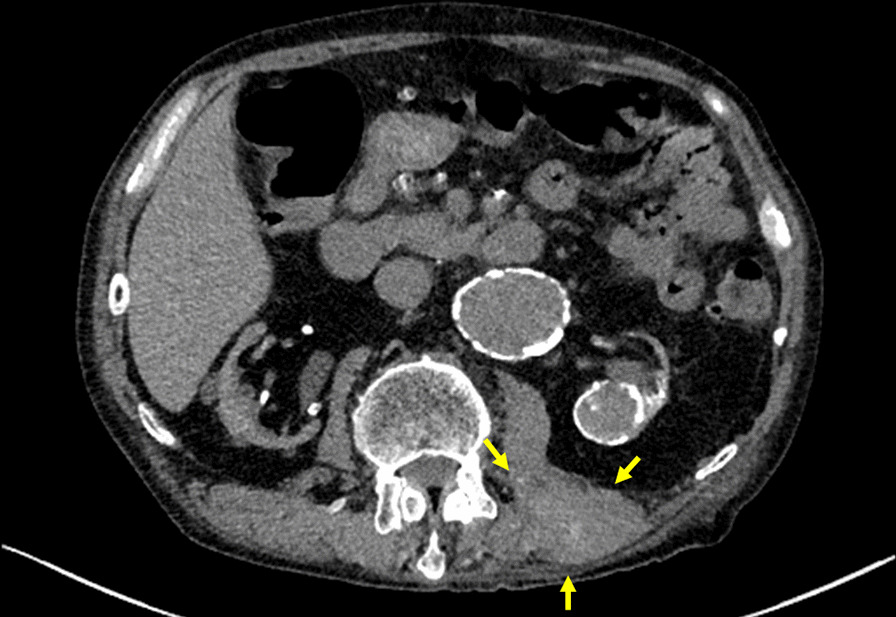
Axial enhanced computed tomography scan images showed a tumor that had spread to the left erector spinae muscle. The lesion appeared to have a nodular structure including high and low attenuation areas (yellow arrow).
